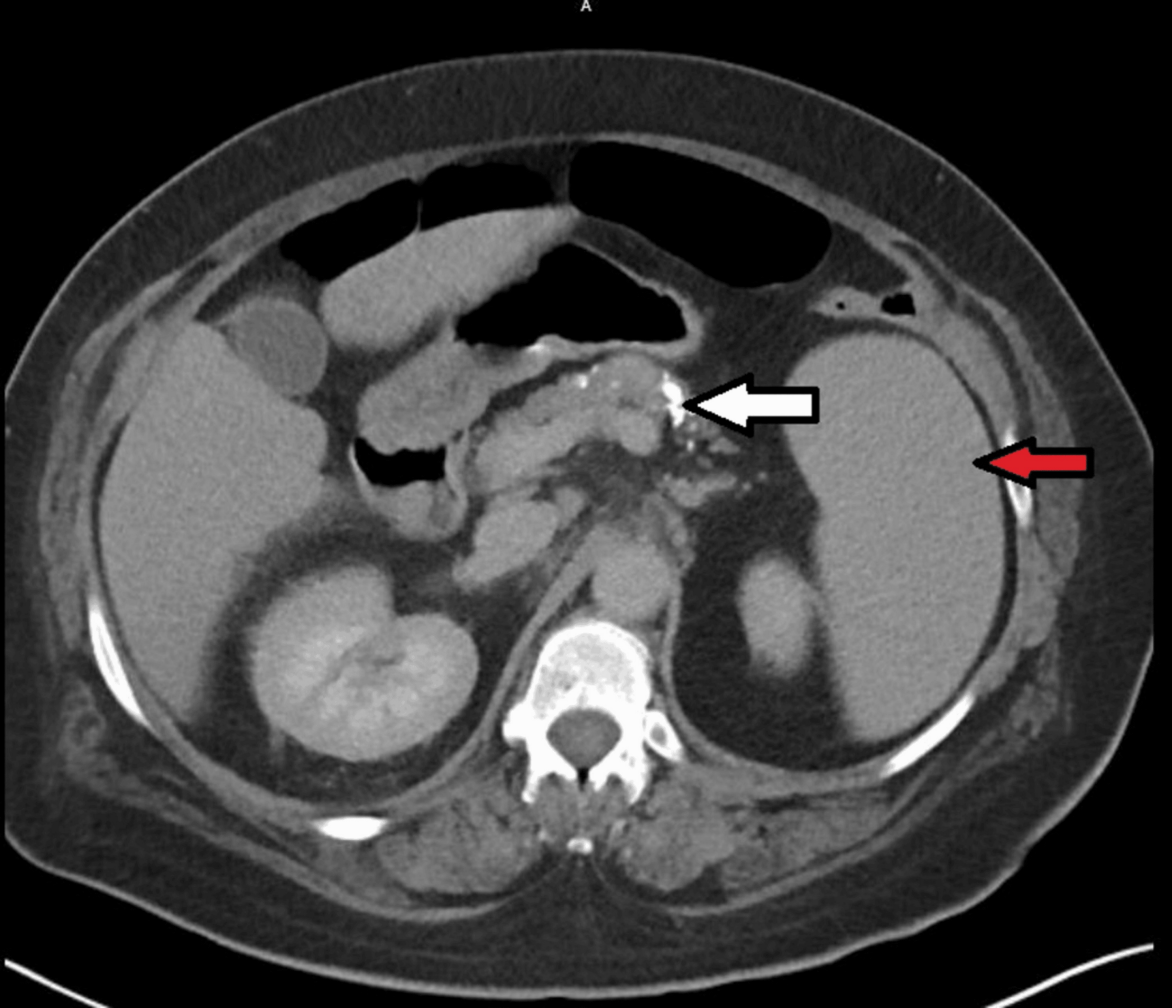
CT of the abdomen and pelvis with contrast revealed chronic pancreatitis (white arrow) and splenomegaly (red arrow).
